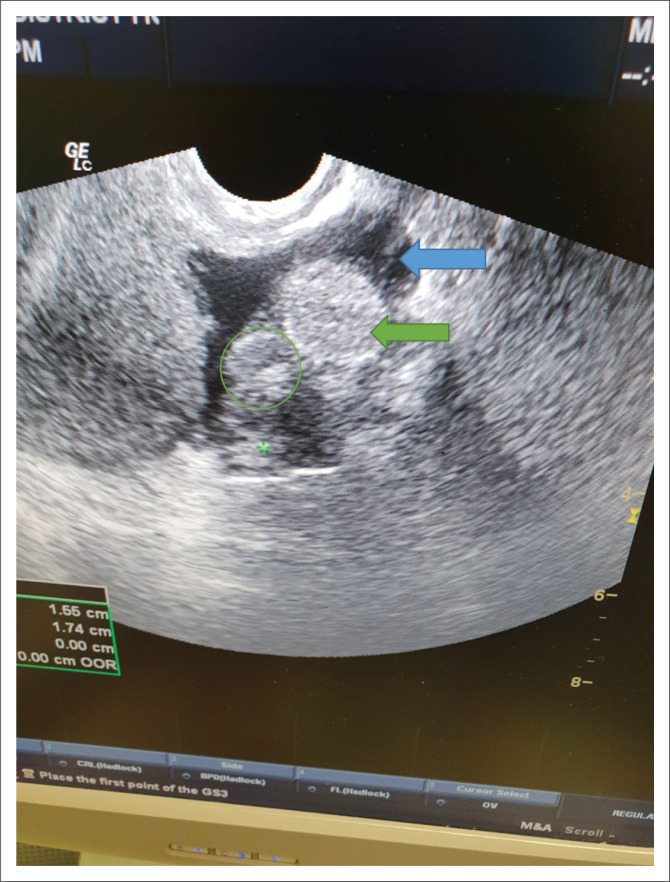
Transvaginal ultrasound showing right adnexal mass (green circle). Note the empty uterus (green arrow) and bladder (blue arrow).
